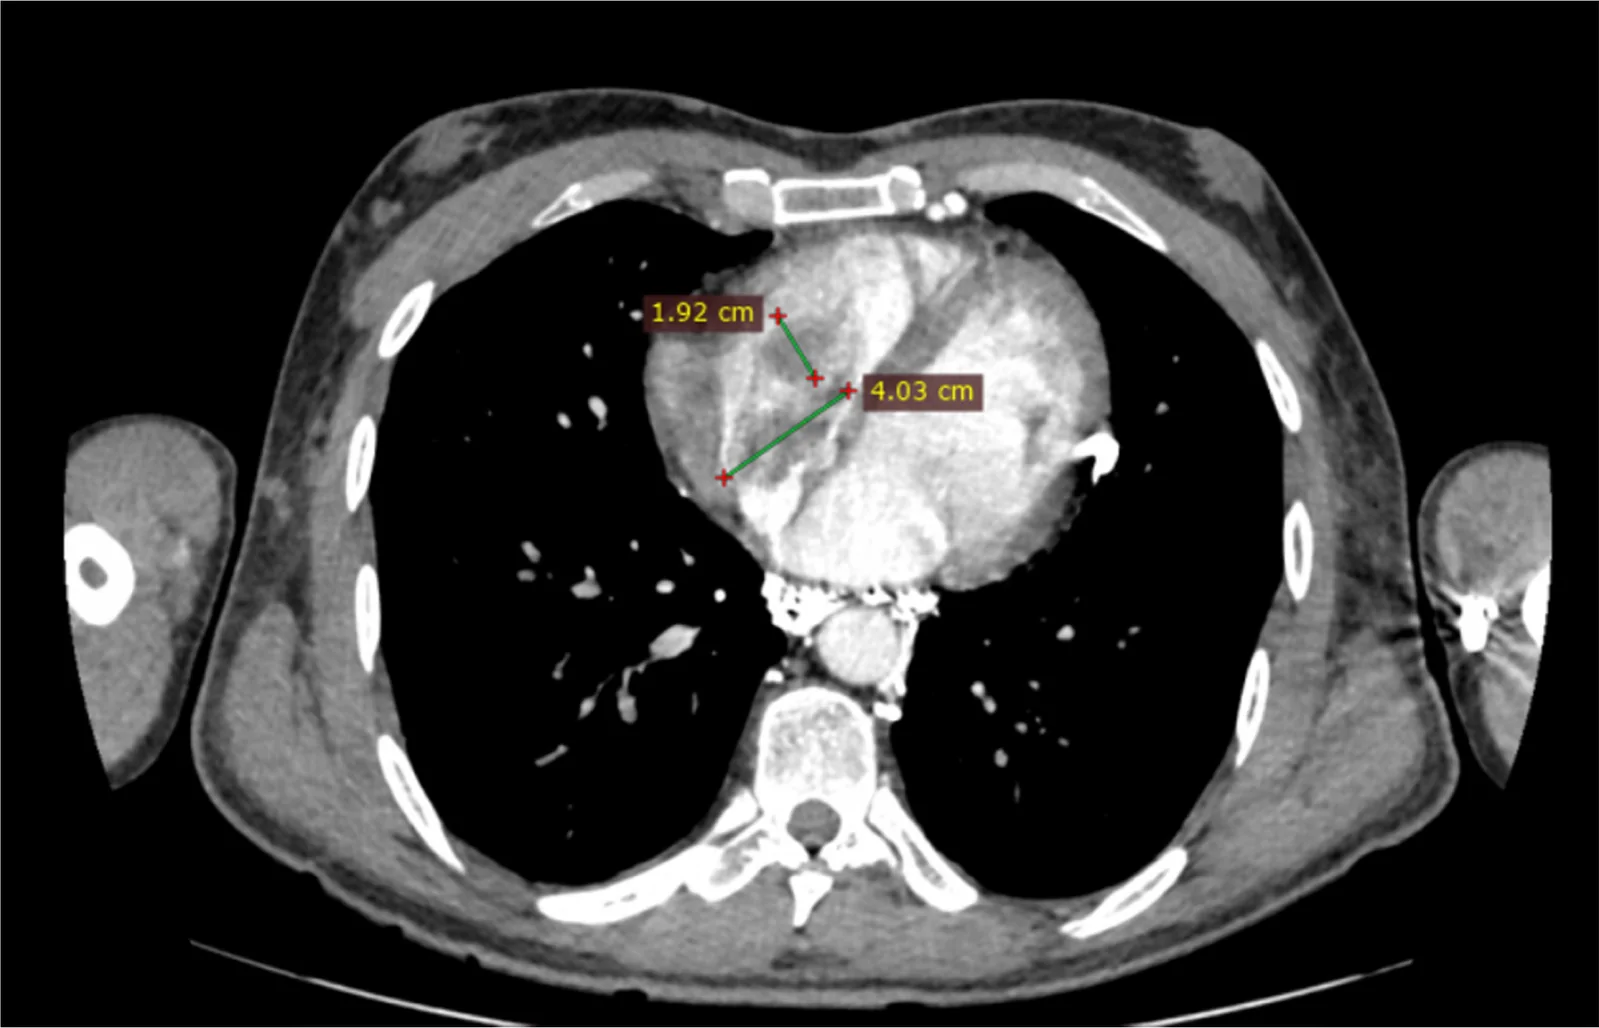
Contrast-enhanced axial chest CT image showing a large hypodense intracavitary filling defect within the right atrium (RA), consistent with thrombus. The lesion measures approximately 4.03 × 1.92 cm (calipers) and appears partially attached to the atrial wall.
